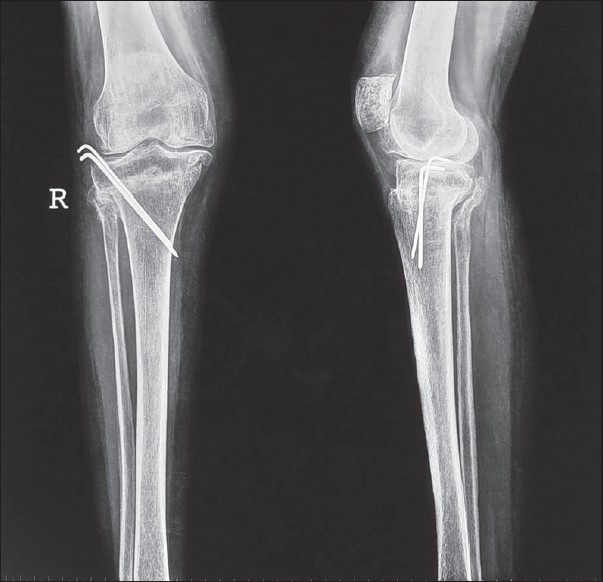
X-rays soon after HTO showing K wires and lateral and posterior ledges from the proximal segment. Note slight anterior positioning of tibial tubercle from the distal fragment
